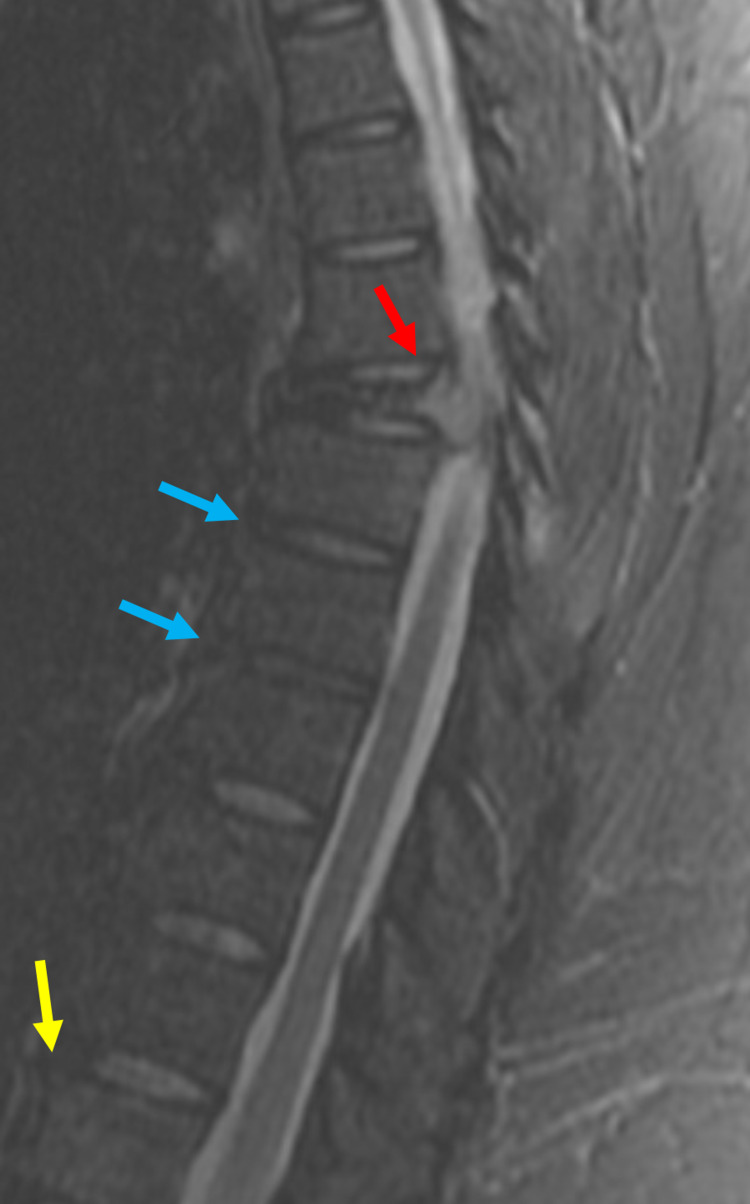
MRI showing loss of height at T6 vertebrae and cord compression (red arrow), demineralization (yellow arrow) at the T12 vertebrae, and diffuse mild spondylosis of the thoracic spine (blue arrows).
